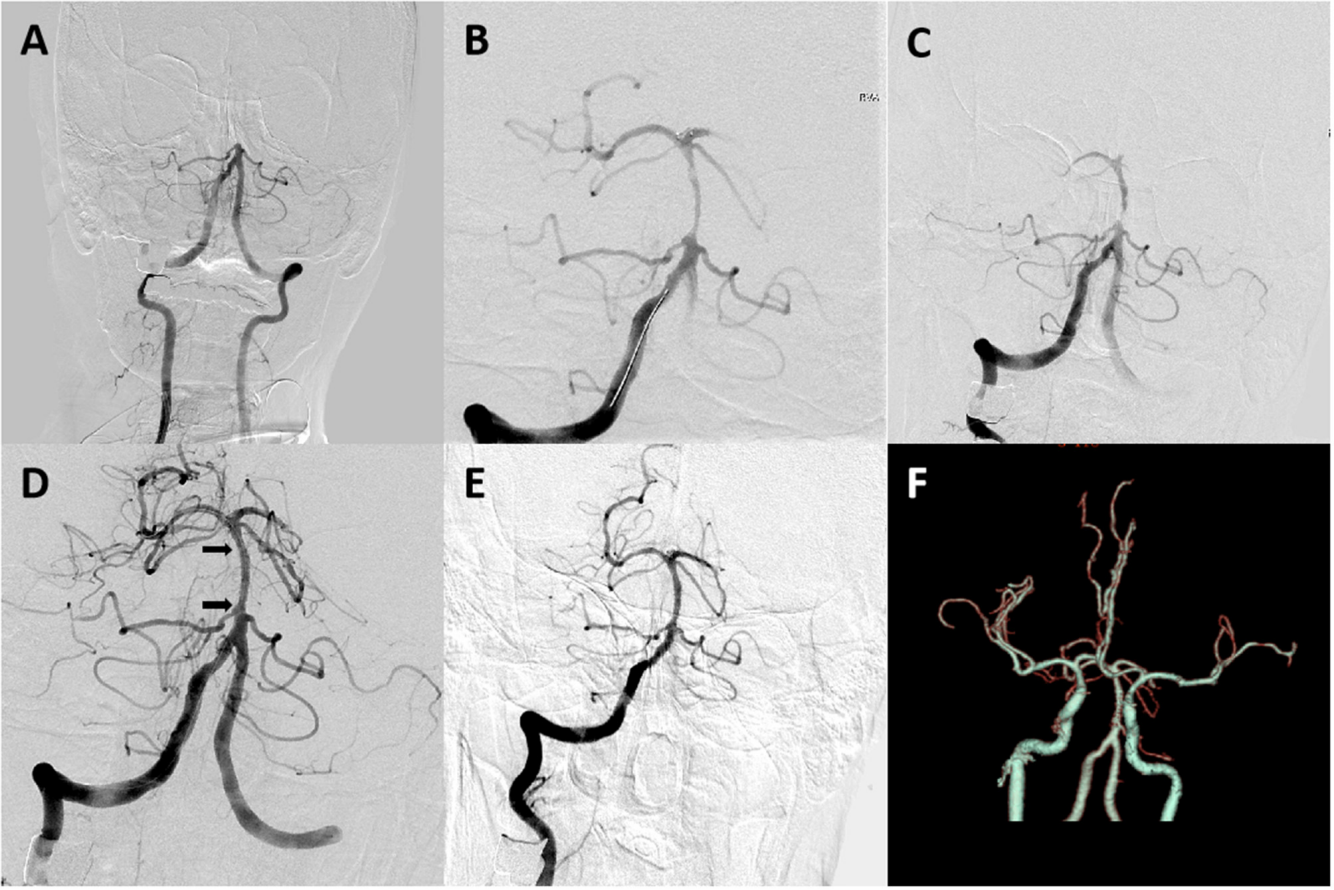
A 62-year-old male patient with coronary artery disease for 20 years and hypertension for 3 years presented with disturbance of consciousness for 4 h. (A) Digital subtraction angiography revealed occlusion at the proximal basilar artery segment. (B,C) Angiogram obtained after one Solitaire AB pass revealed severe underlying atherosclerotic stenosis of the proximal basilar artery segment. (D,E) Digital subtraction angiography after stent placement revealed good perfusion with fixed, focal basilar artery stenosis. Arrows indicate proximal and distal ends of the Apollo stent. (F) CT angiography on 24-h follow-up revealed a patent basilar artery. The patient had a modified Rankin scale (mRS) score of 3 on a 3-month follow-up.
